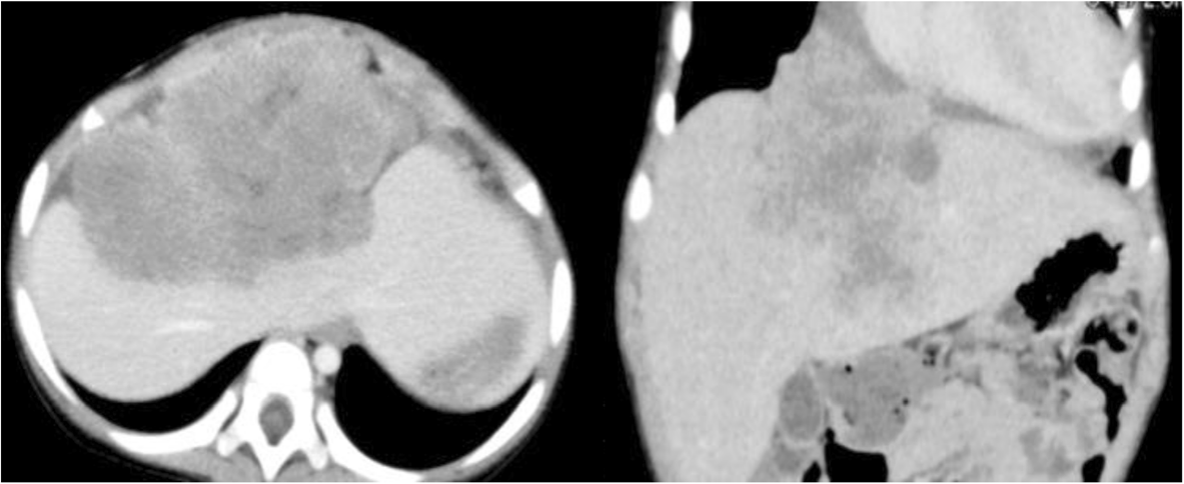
CT images after 6 courses of VAC and 2 courses of ICE, just before the start of PBT
